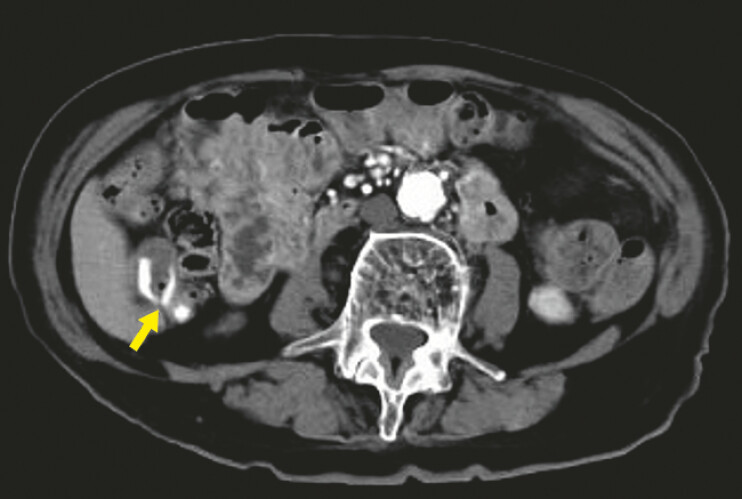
Contrast-enhanced computed tomography revealed extravasation in the ascending colon (arrow).
